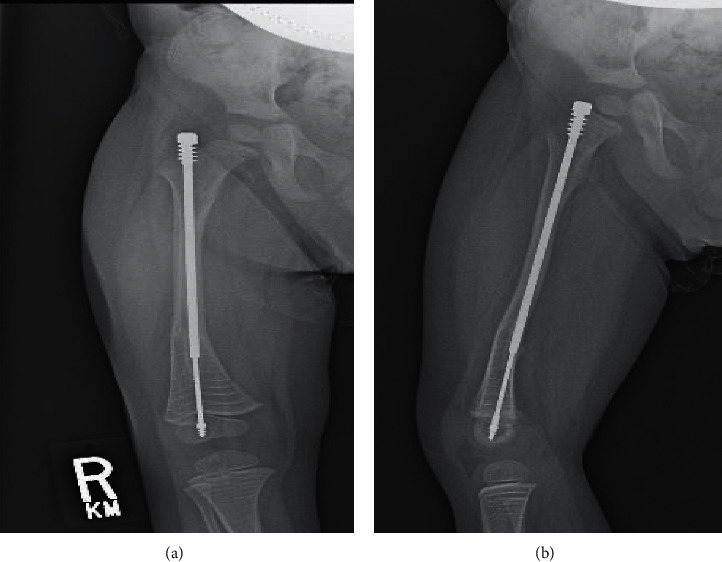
(a), (b) AP and lateral radiographs demonstrating the 3.2 mm Fassier–Duval rod in place with slight bending in the coronal plane.
